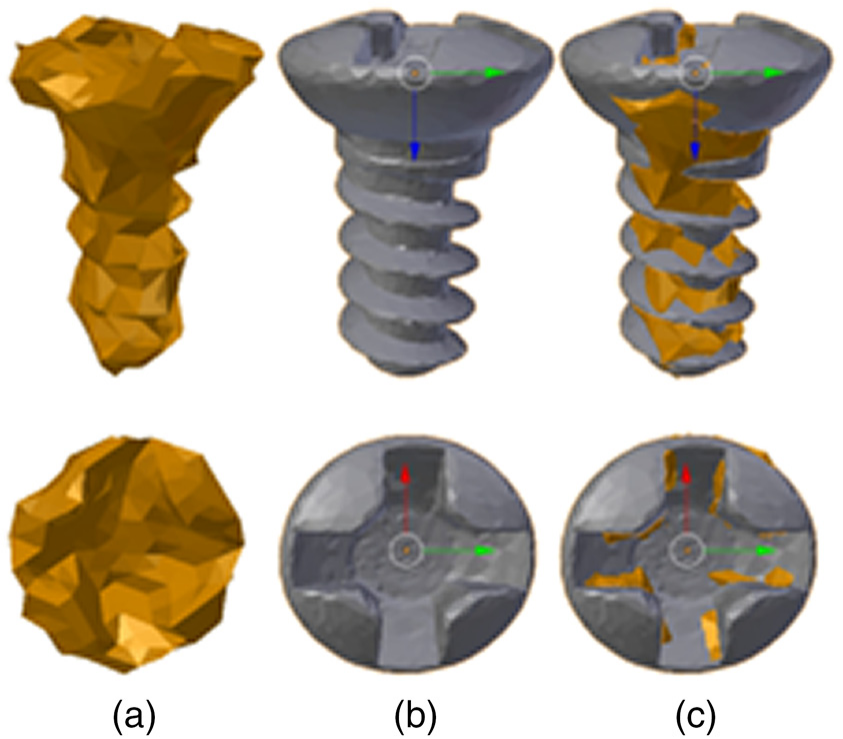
Samples of screw models with screw body and cross-head in upper and bottom figures. (a) A mesh model of the segmented screw in a virtual CBCT image. (b) A mesh model of the reference screw used for the alignment. The bottom of the screw at the cross-head section is defined as a point of reference (marked in x, y, and z axes). (c) Coregistered segmented screw model (a) to the reference model (b).
